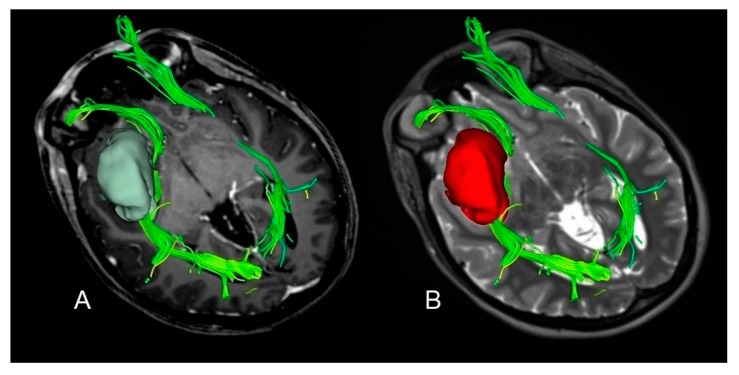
A case of insular diffuse low grade gliomas (DLGG) with a regular shape, determining similar tumor volume in both post-contrast T1-weighted MRI and T2-weighted MRI sequences and displacing the Fronto-Occipital Longitudinal Fasciculus. The preoperative tumor volume computed on post-contrast T1-weighted magnetic resonance imaging (MRI) was 32 cm^3^ (axial slices A). The preoperative tumor volume computed on T2-weighted MRI was 34 cm^3^ (axial slices B). The preoperative ΔT2T1 MRI index was 2 cm^3^, showing the prevalence of the proliferative tumor growing pattern. The patient was in Engel Class IA 18 months after surgery.
